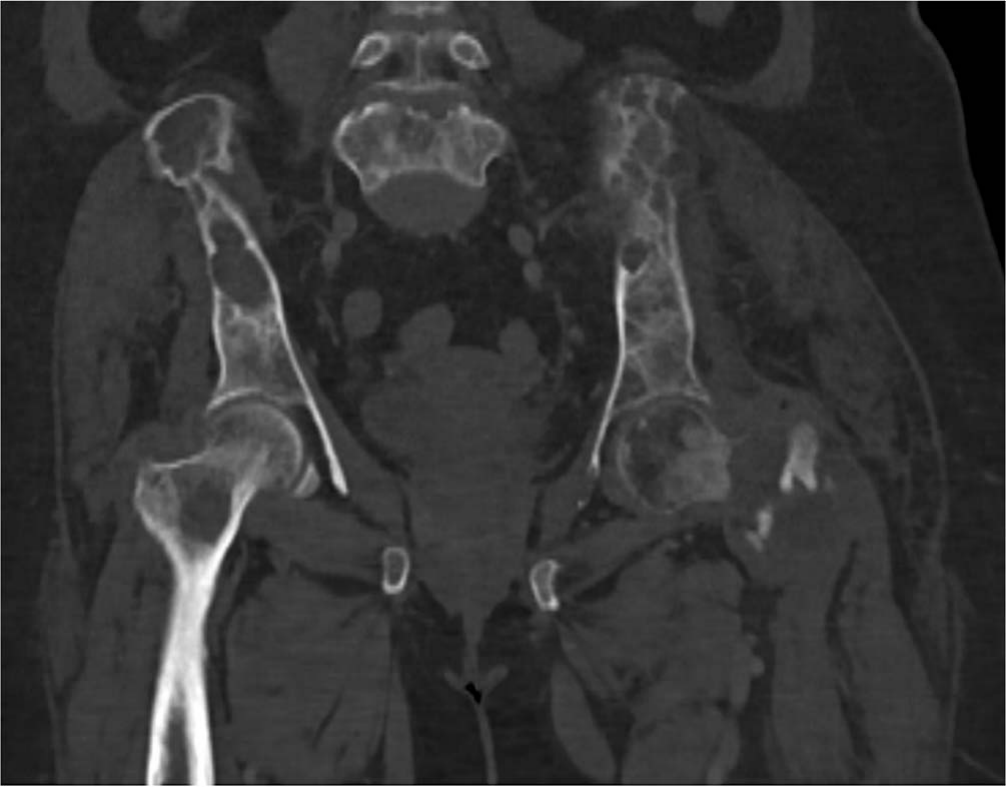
Pelvis CT scan in coronal view, bone window showing diffuse osteopenia of the sacrum and iliac bones with cortical thinning and multiple pathological fractures of the left femoral neck, including transcervical and intertrochanteric components.
